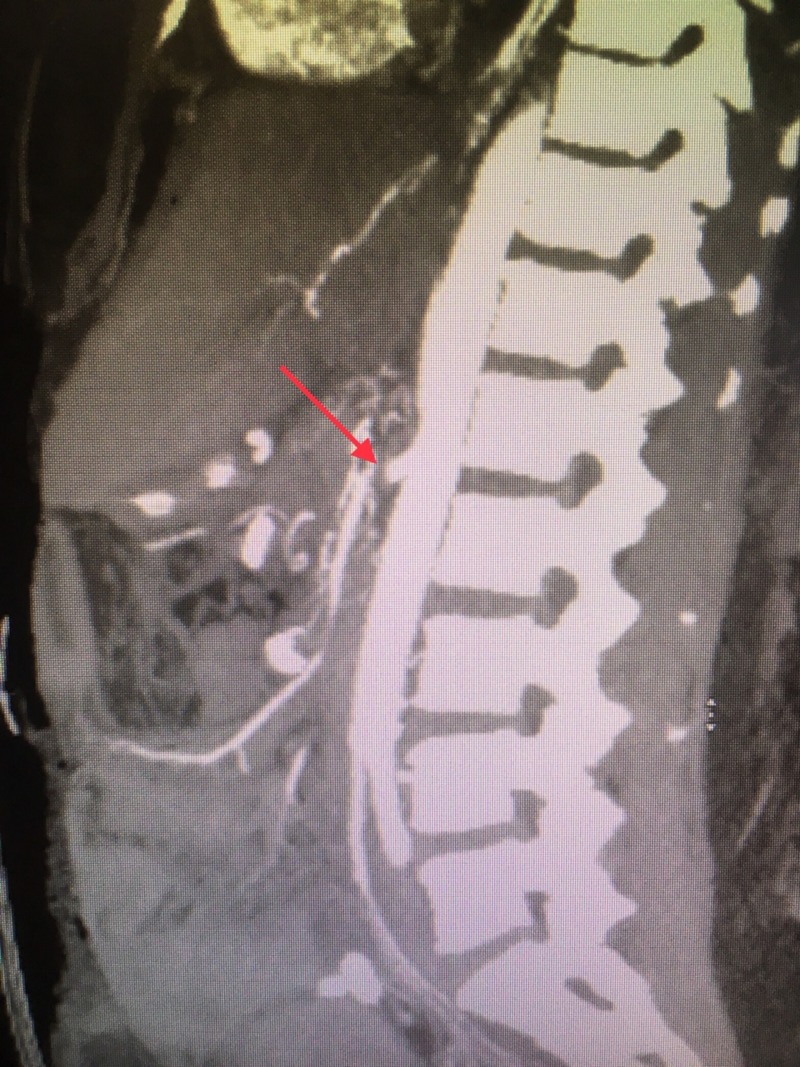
Computed tomography angiogram of the abdomen Superior mesenteric artery occlusion (red arrow)
